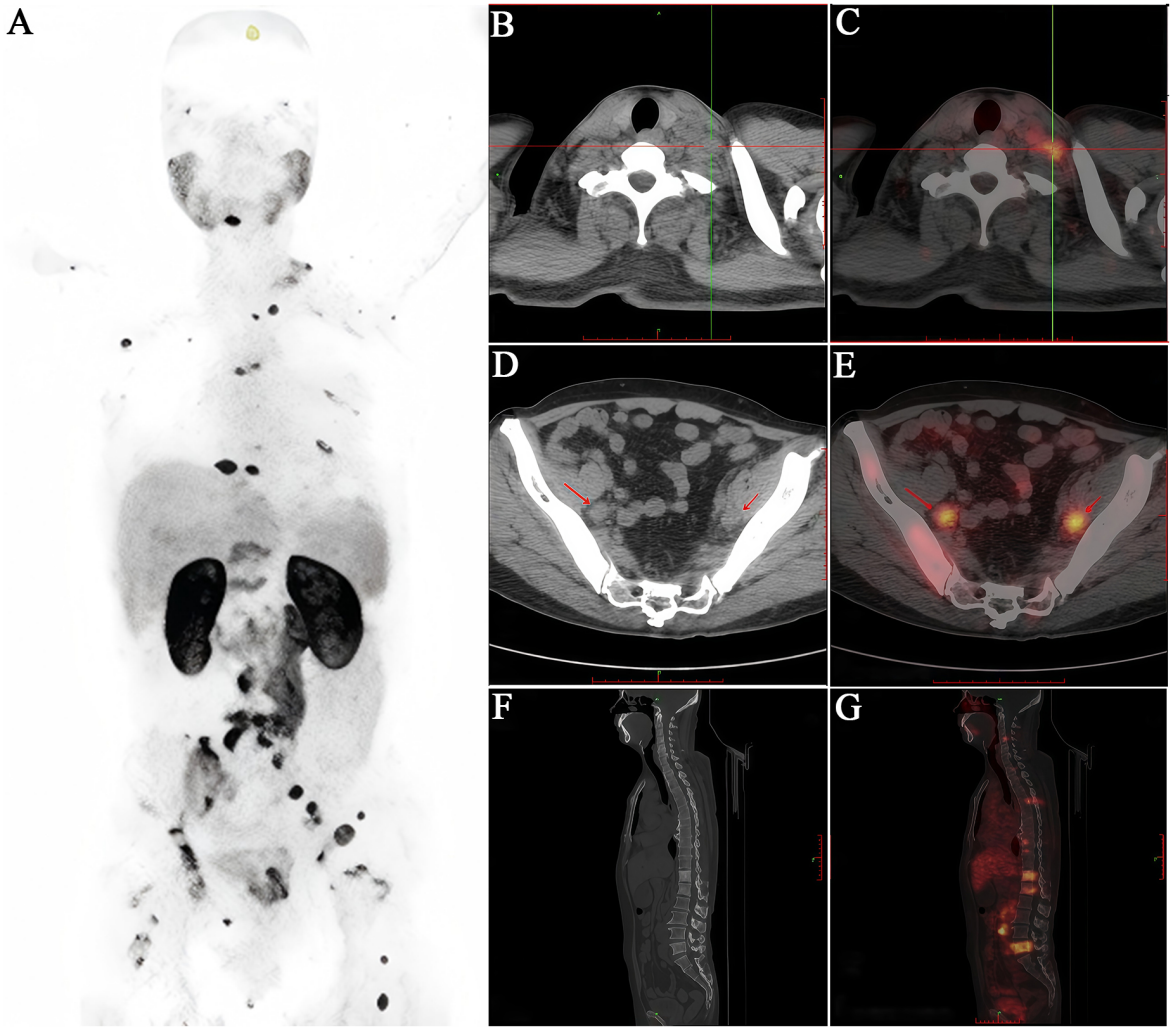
A 65-year-old male patient diagnosed with prostatic acinar adenocarcinoma (Gleason 4+4=8) by biopsy received an intravenous injection of ^18^F-PSMA. (A) PET/CT scan was performed 50 minutes later. (B, C) Multiple lymph nodes of varying sizes were observed in the left neck with increased radioactive uptake to different degrees (SUVmax 3.3). (D, E) Enlarged lymph nodes with increased radioactive uptake were seen around the bilateral iliac vessels (SUVmax 10.2). (F, G) Multiple vertebral bodies and some appendages of the spine, as well as the bones of the pelvis, showed significant bone destruction with increased radioactive uptake (SUVmax 6.7). It can be seen that the ^18^F-PSMA PET/CT scan comprehensively reflects the multiple metastatic burdens throughout the body of this PCa patient, providing a strong basis for clinical decision-making.
